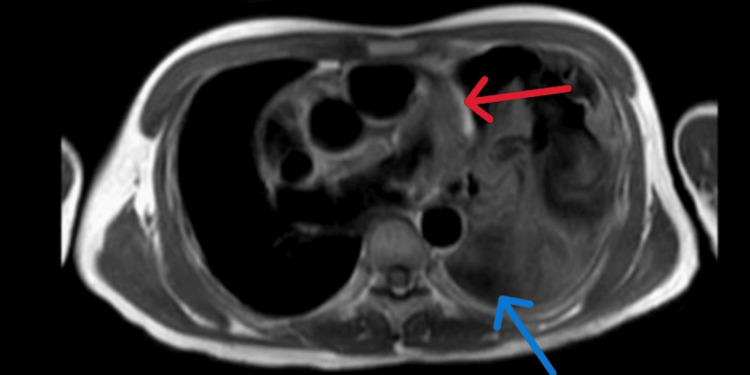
Cardiac MRI (axial view) demonstrating a thickened, enhancing pericardium (red arrow) without pericardial effusion, and a left-sided pleural effusion (blue arrow).
